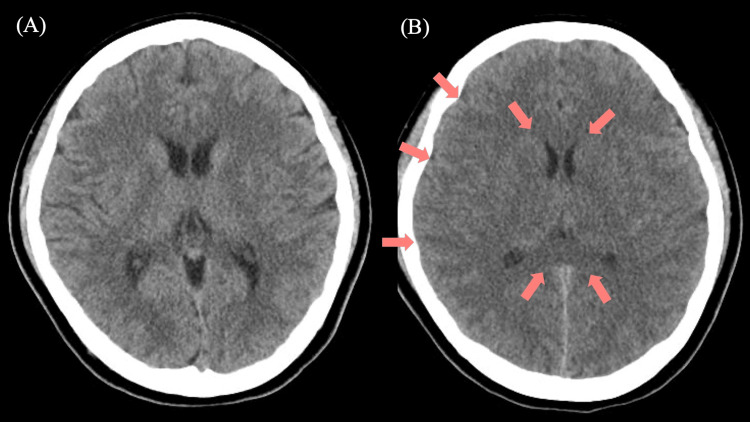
Brain computed tomography (CT) upon admission and on day 5 of admission. (A) Non-contrast axial brain CT upon admission showing no acute abnormalities. (B) Non-contrast axial brain CT on day 5 demonstrating diffuse cerebral edema with effacement of cortical sulci and ventricles, and subtle loss of gray-white matter differentiation (arrows).
